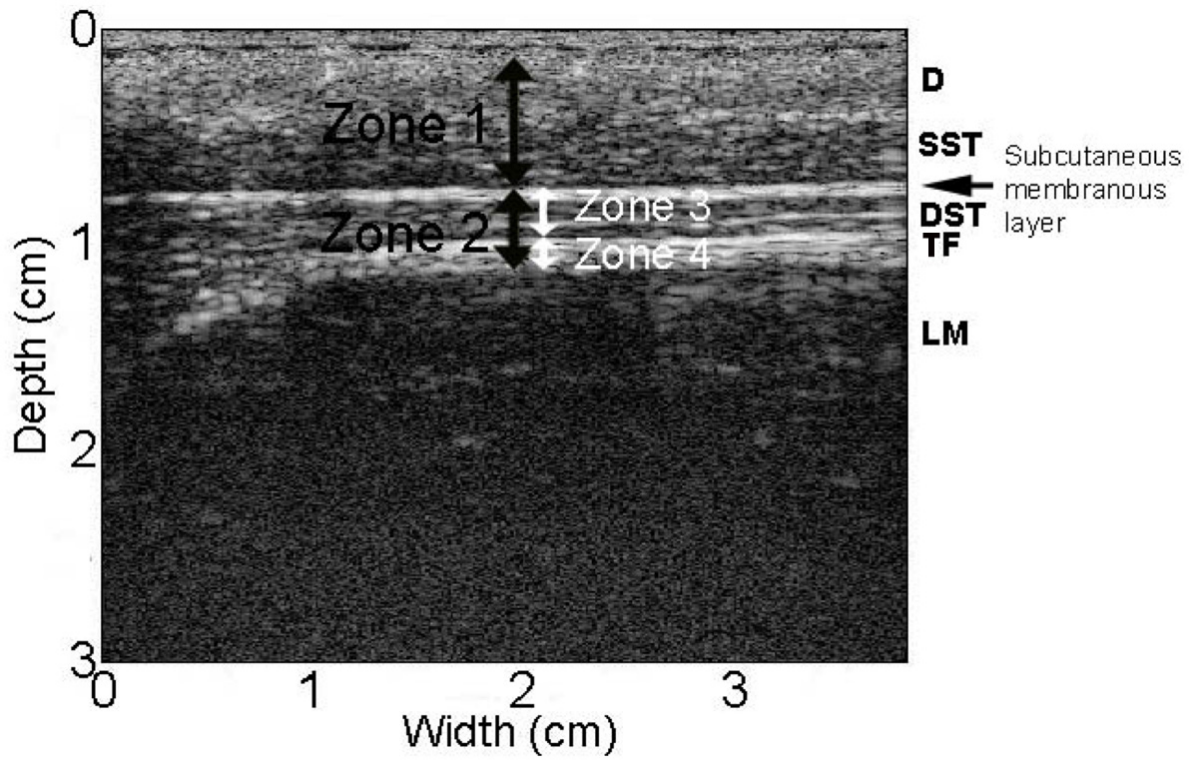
Location of tissue zones used for measurement of tissue thickness in ultrasound images. D: dermis, SST: superficial subcutaneous tissue, DST: deep subcutaneous tissue, TF: thoracolumbar fascia.
